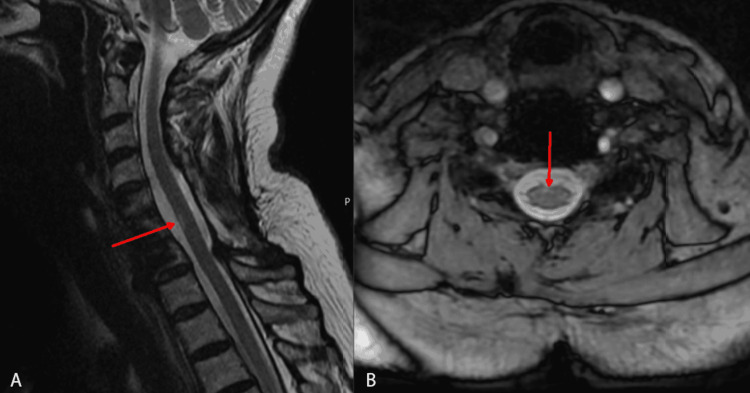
MRI-T2 image at 21 months post-operatively. A: Sagittal view, the red arrow indicating the small focus of myelomalacia along the anterior median sulcus of the cord at the C6 level; B: Axial view, red arrow again indicating myelomalacia of cord at C6.
